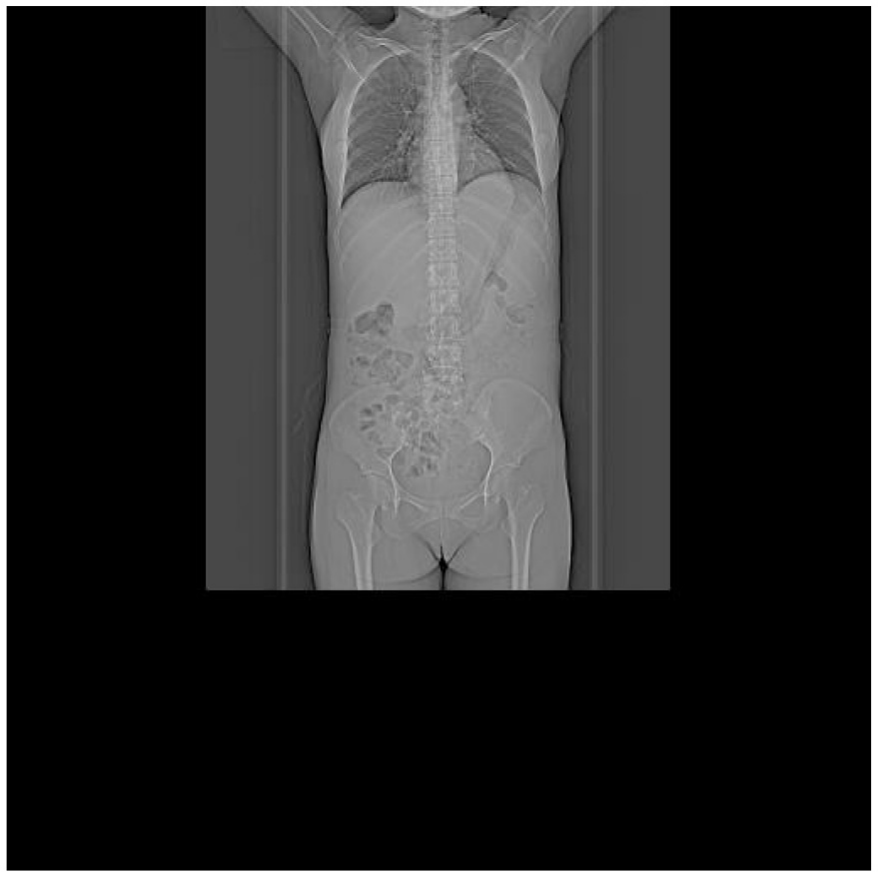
(C) PET-CT at admission (25 February 2025)	(D) PET-CT at admission (25 February 2025)
